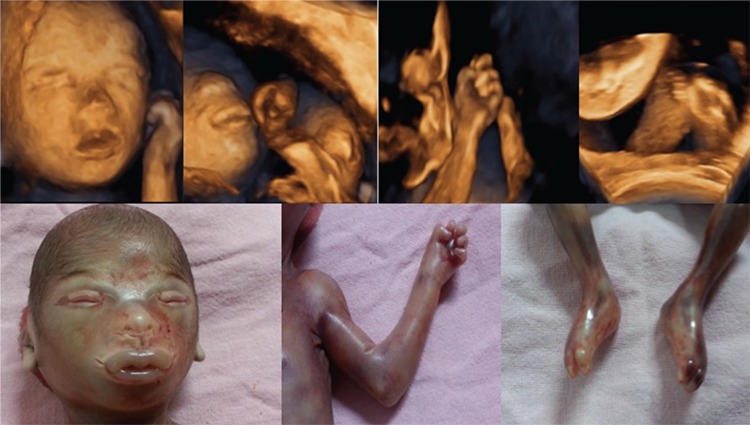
Antenatal 3-dimensional-ultrasound images and post-natal images of the fetus in the second pregnancy showing the typical phenotypic features of Harlequin ichthyosis (eclabium, ectropion, small nose, clenched fists and incurved toes)
